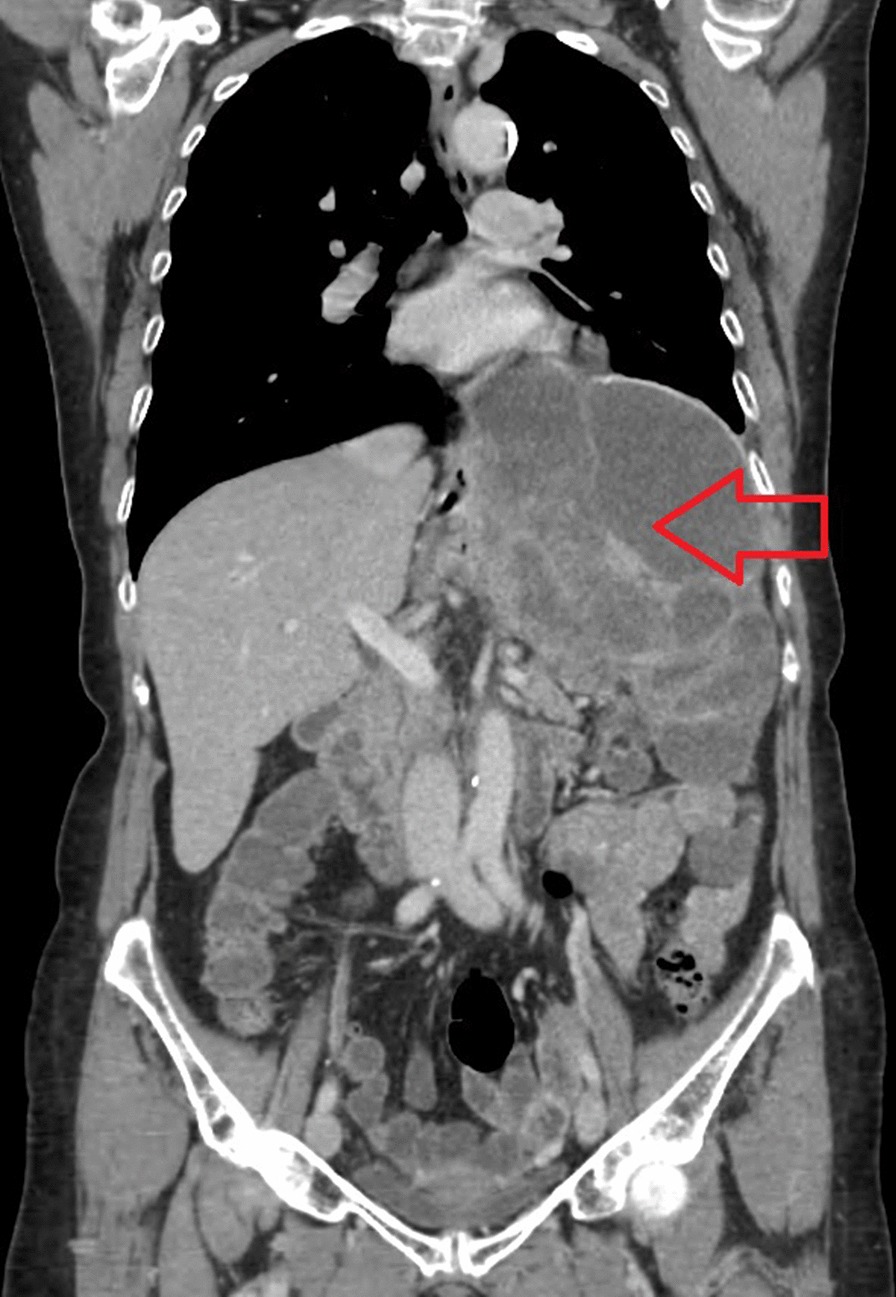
CT-frontal view of recurrent tumor at the site of the spleen, 3 years after surgery (red arrow)
